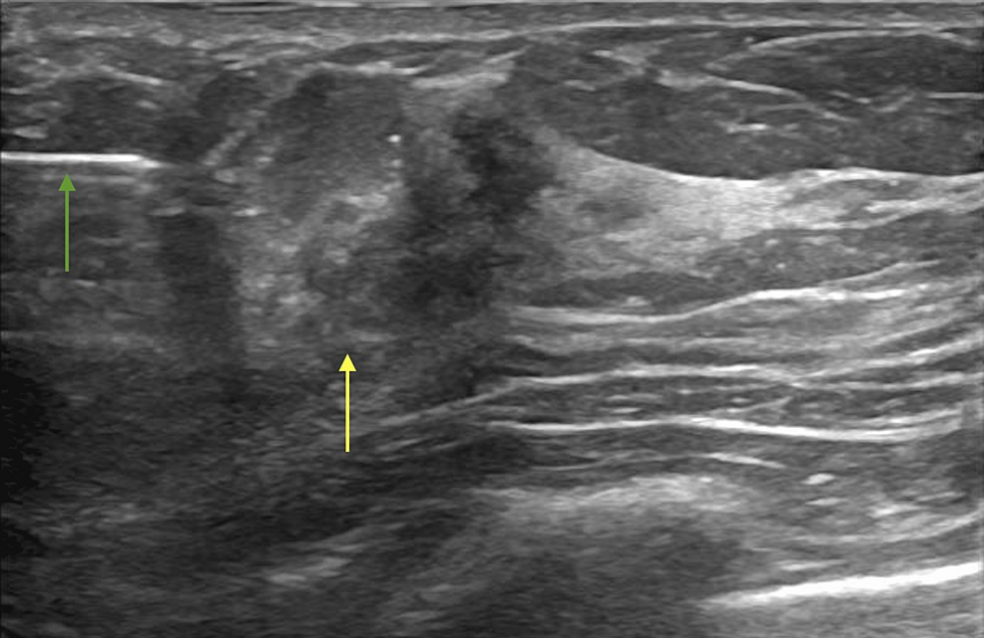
Ultrasound-guided biopsy of the 10 o'clock mass Green arrow: biopsy needle Yellow arrow: mass
